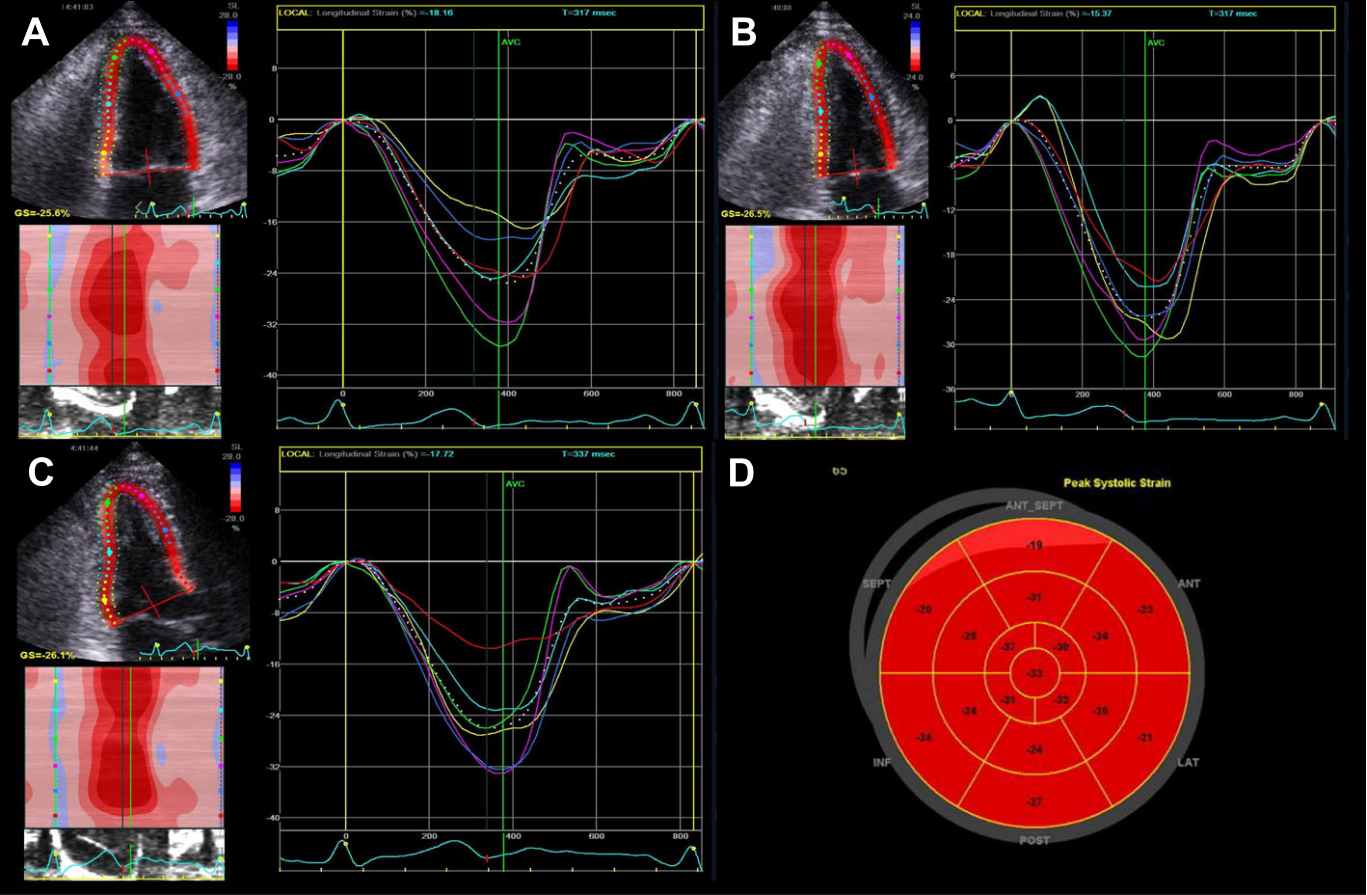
Demonstration of a 2-dimensional strain analysis with GE EchoPAC PC software. After tracing of the endocardial border, the software provides global and regional myocardial strain values automatically in apical 4 chamber (A), apical 2 chamber (B), and apical 3 chamber views (C). The GE EchoPAC algorithm can provide bull’s eye maps of regional longitudinal strain values (D)
